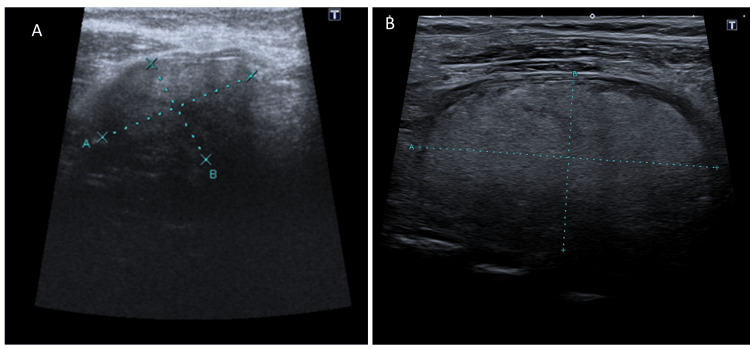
The thyroid ultrasound revealed a notable nodule (EU-TIRADS 4) measuring 45x28 mm in the right lobe (A), and its evolution after four years (B), measuring 59x33 mm. (anteroposterior × width) EU-TIRADS: European Thyroid Imaging Reporting and Data System
